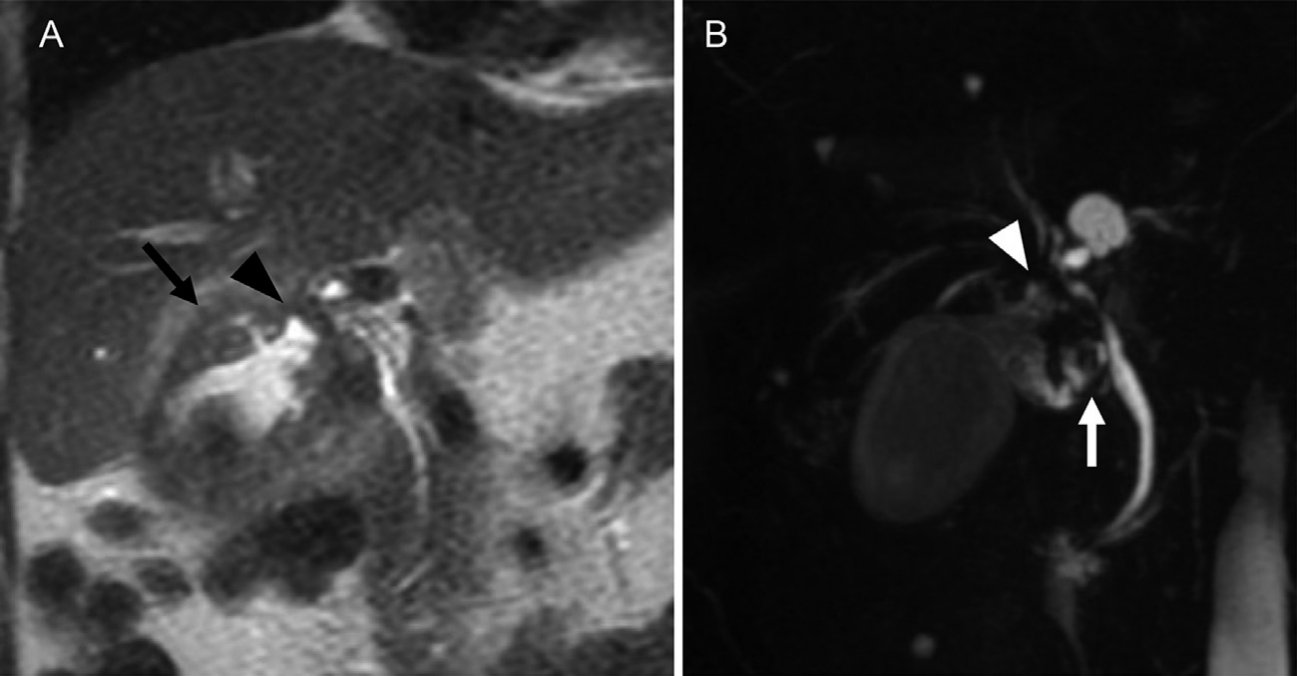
(A) Coronal image of T2-weighted MRI shows pericholecystic fluid collection (arrowhead) and wall thickening of the gallbladder (arrow). (B) MRCP shows incarcerated stone (white arrow) and pericholecystic fluid collection (white arrowhead). MRI, magnetic resonance imaging; MRCP, magnetic resonance cholangiopancreatography.
